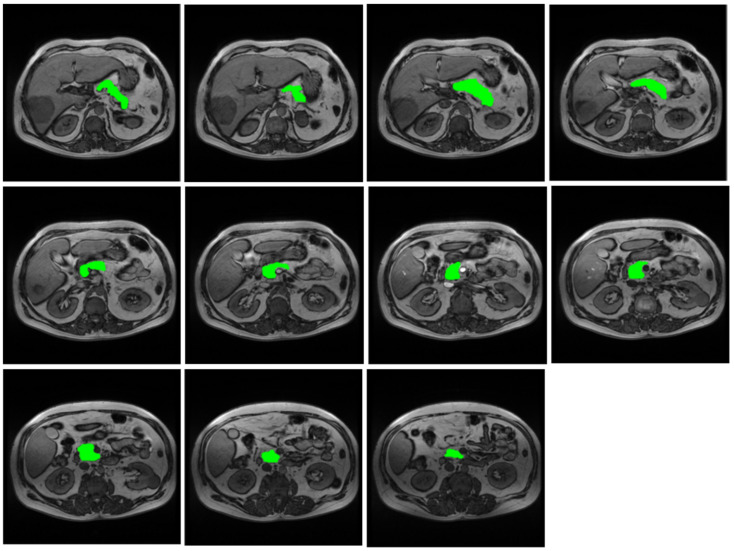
Procedure for the evaluation of pancreatic volume from axial T1-weighted GRE MRI images. A region of interest encompassing the pancreatic parenchyma was manually traced in each slice and its cross-sectional area was calculated. All such measurements through the pancreas were multiplied by the slice thickness and summed to calculate the pancreatic volume.
